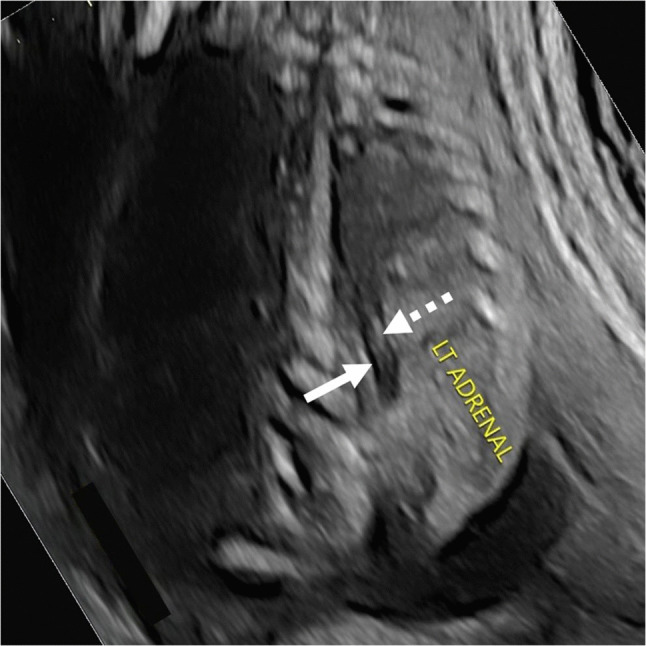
Coronal ultrasound imaging of a 23-week female fetus with left renal agenesis demonstrating the abnormal configuration of the adrenal gland, which has a laying, linear configuration, rather than the usual inverted “Y” configuration. Notice the hypoechoic cortex (*white arrow*) and hyperechoic (*dotted white arrow*) medulla. The adrenal gland should be distinguished from the diaphragm (not shown), which is uniformly hypoechoic
